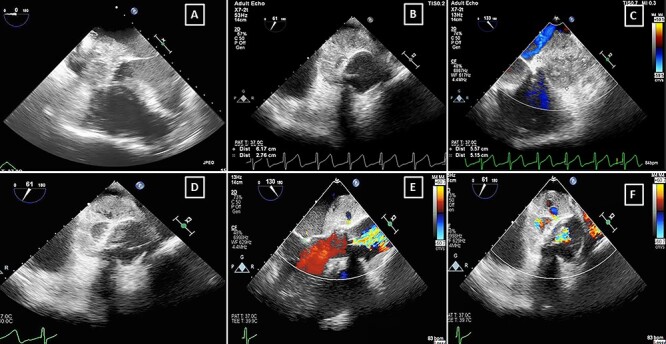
TOE showing the large vacuolated aortic root abscess (A) four-chamber view, (B) at 61°, (C) 133° with colour Doppler, (D) 61° short axis, (E) 130° short axis with colour Doppler showing systolic flow through the abscess indicating fistula formation with the aortic root, (F) 61° Short axis with colour Doppler showing the communication of the aortic root abscess to the aorticroot.
